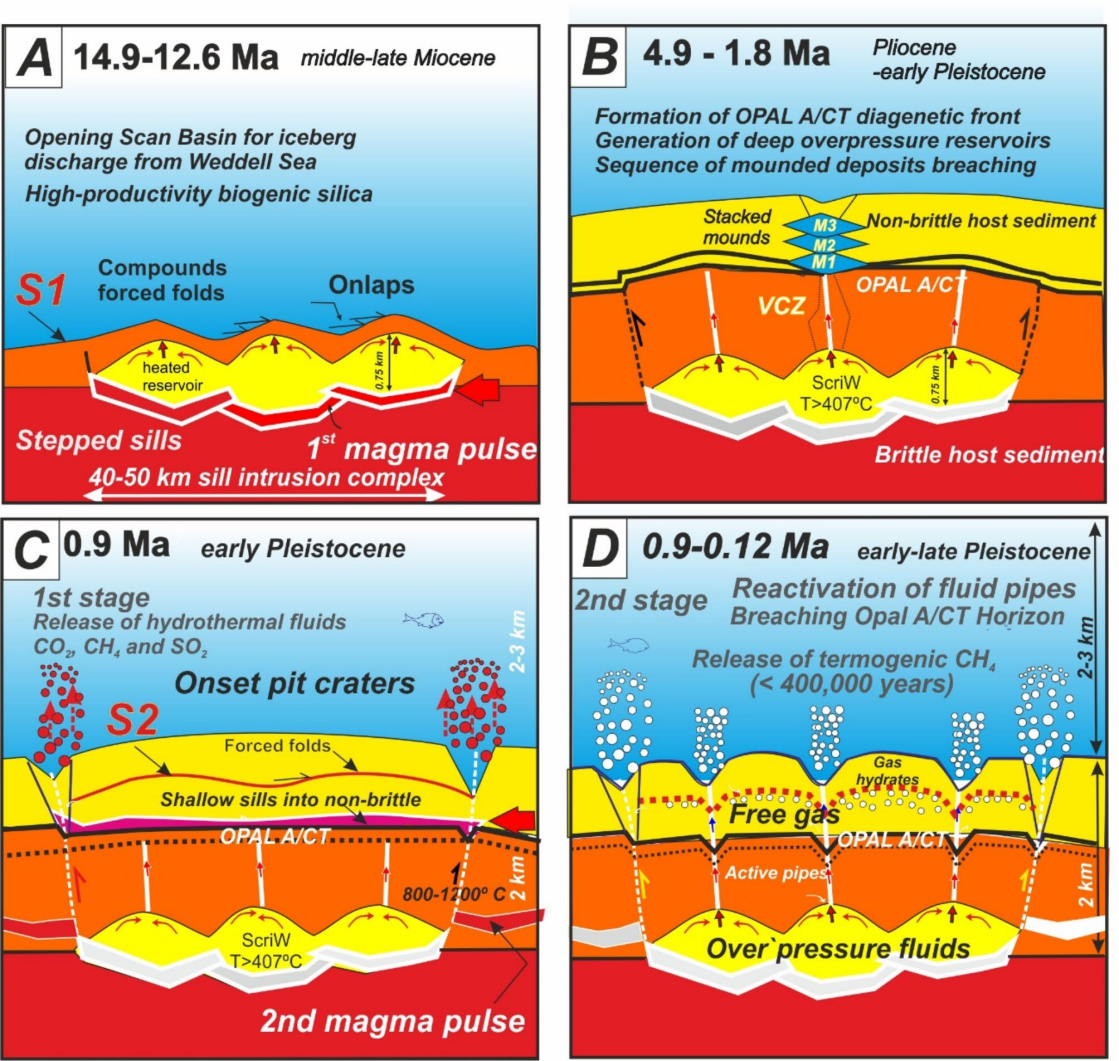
Proposal of evolutionary model of the sill-induced buried hydrothermal system. (A) Magma emplacement during the 1st magma pulse at ca. 14.9–12.6 Ma (middle-late Miocene) generated forced folds above sills by uplift deformation. Continuous release of thermogenic gas from cracking trapped beneath the forced fold-induced domesformed huge thermogenic reservoirs. (B) Opal diagenetic front sealed these reservoirs under conditions of supercritical waters within the buried hydrothermal system^60^. Focused breaching of the opal A/CT Horizon triggered stacked mounds by focused fluid venting above this horizon estimated to occur between horizon-b and -a (4.9–1.8 Ma, Late Miocene-Early Pliocene) according Pérez et al.^57^; (C) A second magma pulse at ca. 0.9 Ma triggered the fluid pipes to the surface forming the sequence of V-shaped craters and (D) Fluid migration sourced from the deep thermogenic reservoirs took at successive reactivation allowing to reach to the surface. Further explanation in the text.
